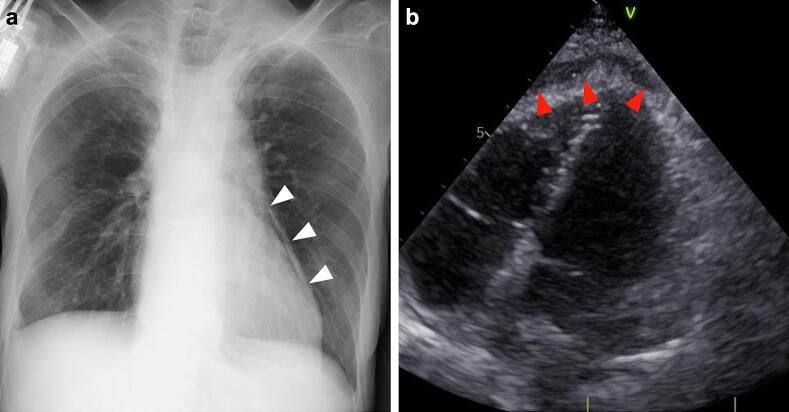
Examinations at the time of emergency. (a) Plain radiography revealed pneumopericardium (white arrowheads). (b) Echocardiography showed pericardial effusion (red arrowheads). (For interpretation of the references to colour in this figure legend, the reader is referred to the web version of this article.)
